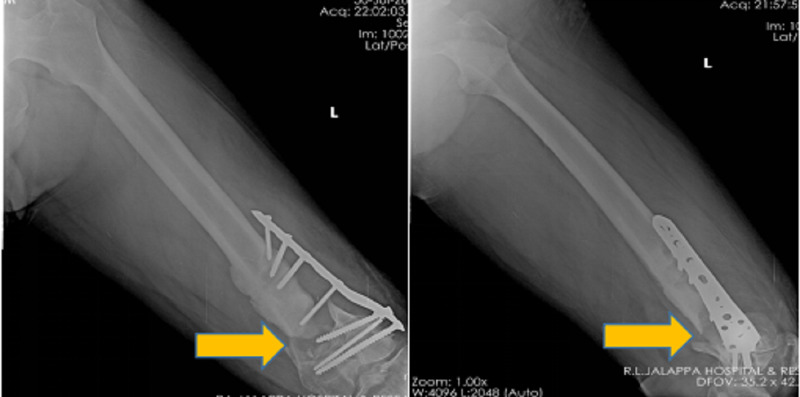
Plain antero-posterior and lateral left thigh radiograph, indicating non-union of left distal femur with locking compression plate in situ.
